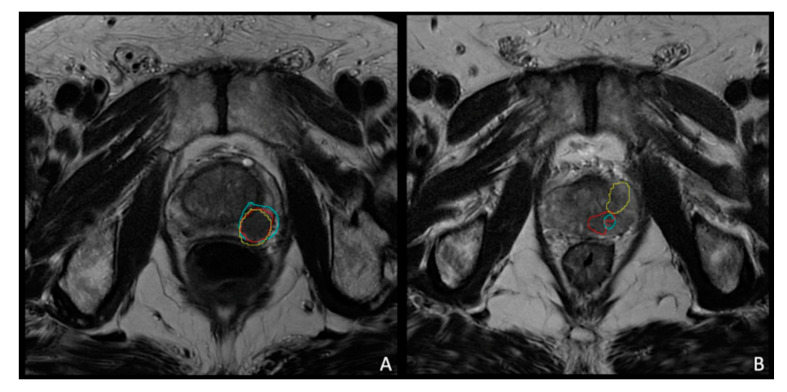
Images representing concordant (A) and discordant (B) contouring on DICE analysis. (A): A 74 years-old patient with biopsy-proven PCa (pt n. 9), Gleason score 9 (5 + 4) with a PSA level at diagnosis of 6.37 ng/mL presenting a prostatic lesion located in the left lobe of the gland. The image shows a concordant identification of the lesion on ^68^Ga-PSMA PET images (blue), ^68^Ga-DOTA-RM2 PET images (yellow) and MRI (red). DICE SCORE: ^68^Ga-PSMA vs. ^68^Ga-DOTA-RM2 = 0.6019, ^68^Ga-PSMA vs. MRI = 0.7581. ^68^Ga-DOTA-RM2 vs. MRI = 0.7220. (B): A 52 years-old patient with biopsy-proven PCa (pt n. 14), Gleason score 8 (4 + 4) with a PSA level at diagnosis of 8.04 ng/mL presenting a focal left prostatic. DICE SCORE: ^68^Ga-PSMA vs. ^68^Ga-DOTA-RM2 = 0.2918, ^68^Ga-PSMA vs. MRI = 0.6162. ^68^Ga-DOTA-RM2 vs. MRI = 0.2216.
